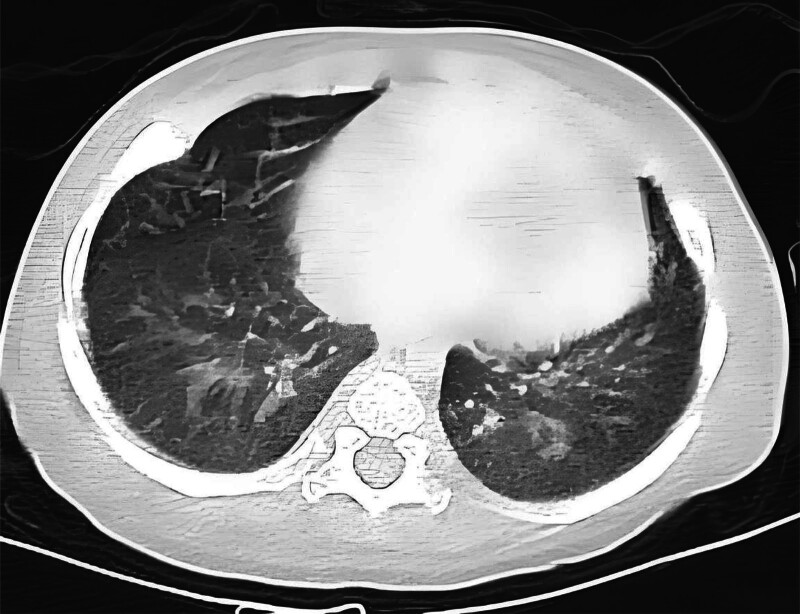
Lung HRCT showed diffuse ground-glass opacity, small amount of fluid in the interlobular pleural space. HRCT = high-resolution computed tomography.
